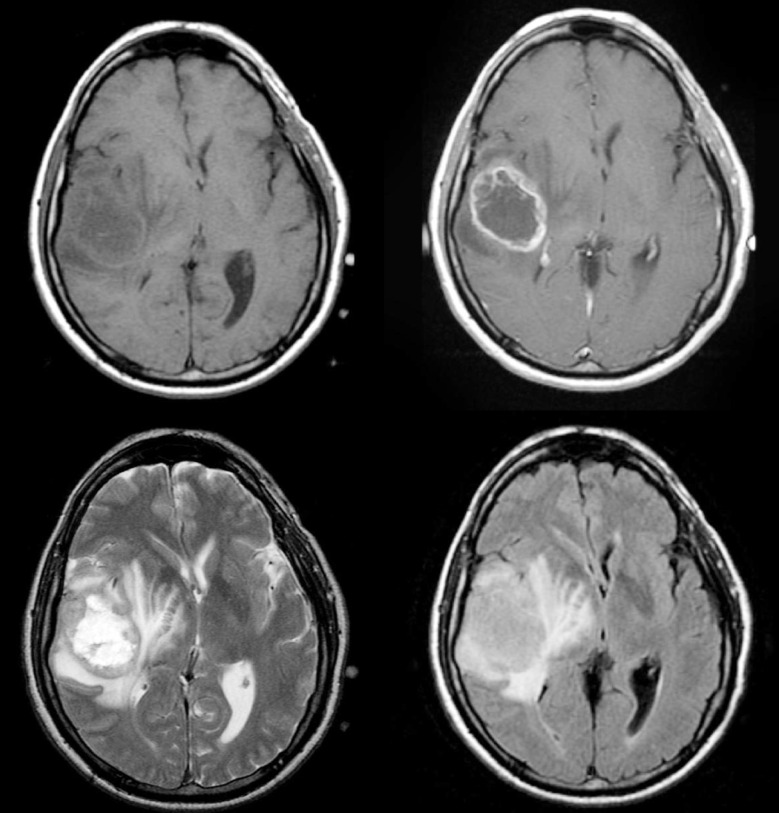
MRI of glioblastoma. A large mass in the right parietotemporal lobe causing significant mass effect with adjacent vasogenic oedema. It appears heterogeneously low on T1, high on T2 and irregular peripheral nodular enhancement. It has a central non-enhancing necrotic component.
